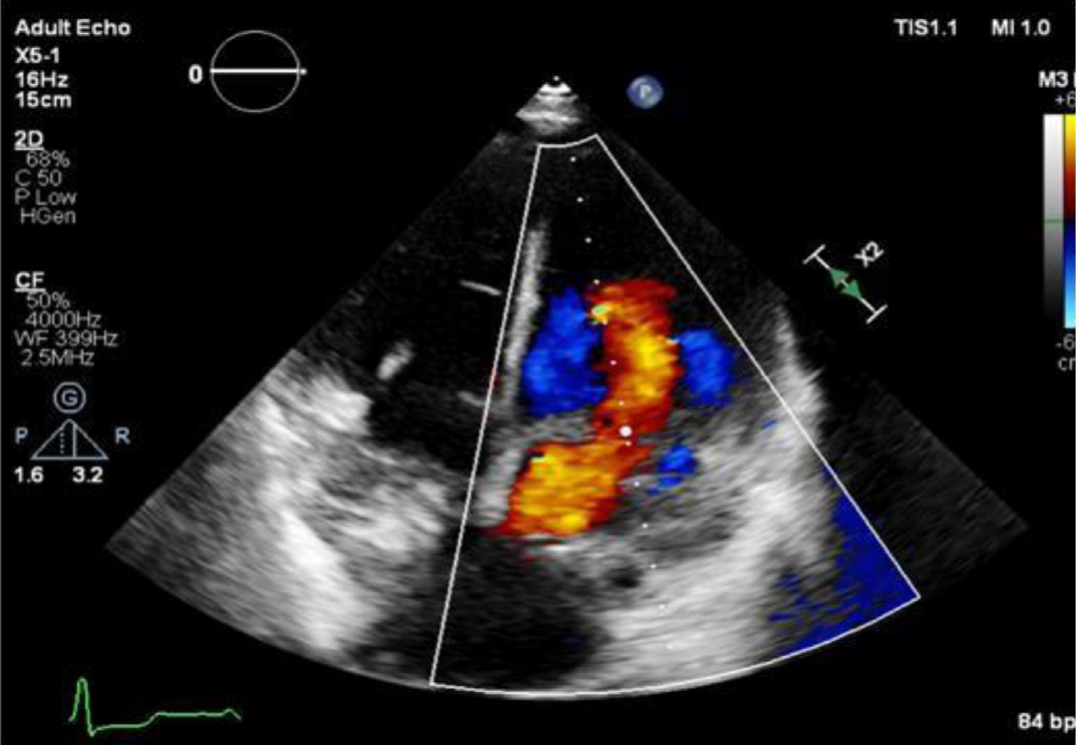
Transthoracic Echocardiography Apical 4-chamber view postoperatively shows the flow across the left atrium with almost no aliasing.
